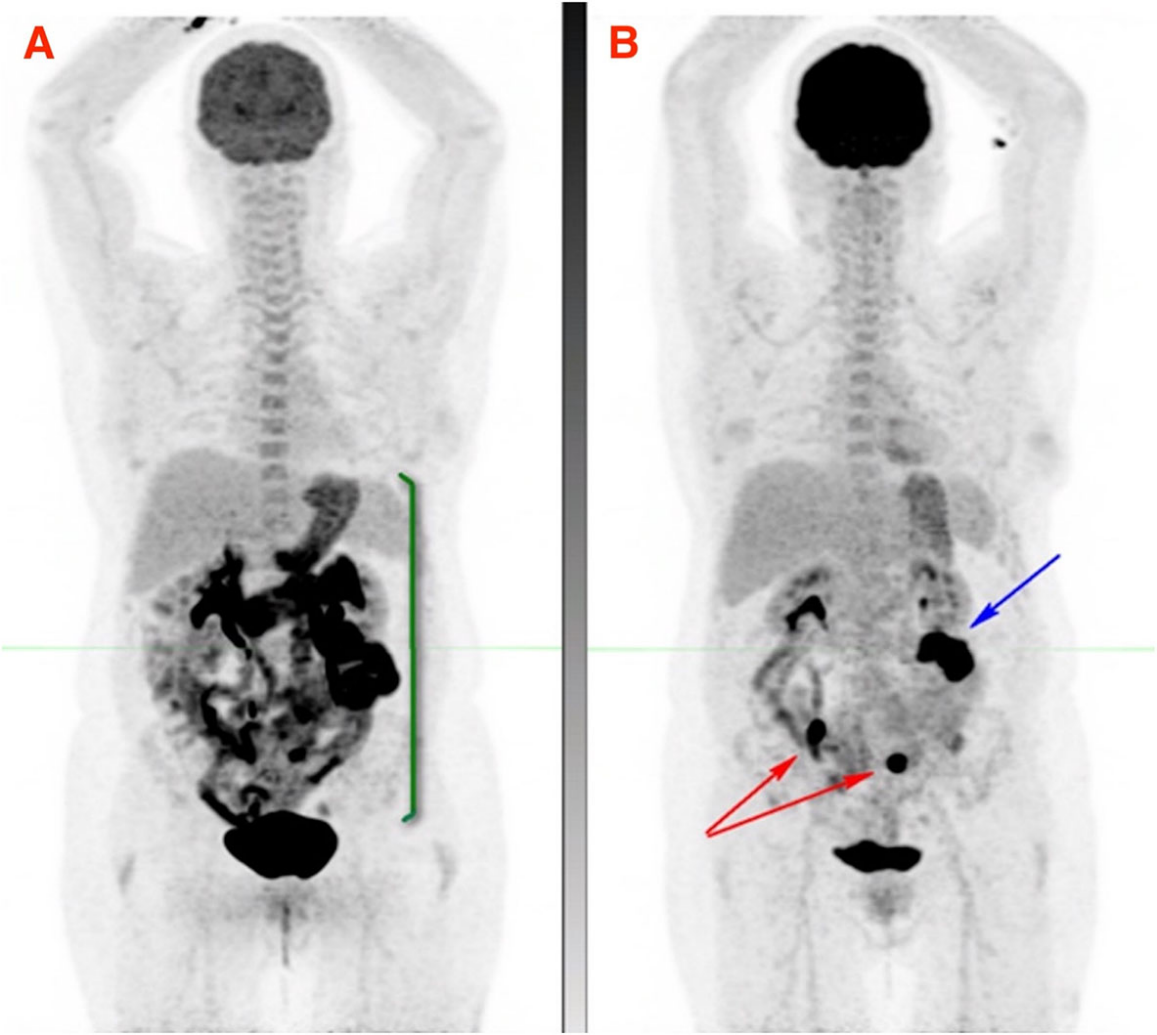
Patient with stage 4 melanoma in CMR on single-agent nivolumab with symptoms of intermittent nausea, vomiting and diarrhoea and clinical diagnosis of immune-related enteritis underwent ^18^F-FDG PET which showed moderate to intense diffuse uptake in the stomach, duodenum and small bowel in keeping with immune-related gastritis, duodenitis and enteritis (MIP image A). Short interval ^18^F-FDG PET after successful treatment of irAEs (MIP image B), showed two foci of intense FDG uptake in the small bowel (red arrows) in keeping with metastases and a third focus in the left abdomen near the transverse colon, in keeping with serosal metastasis (blue arrow)
